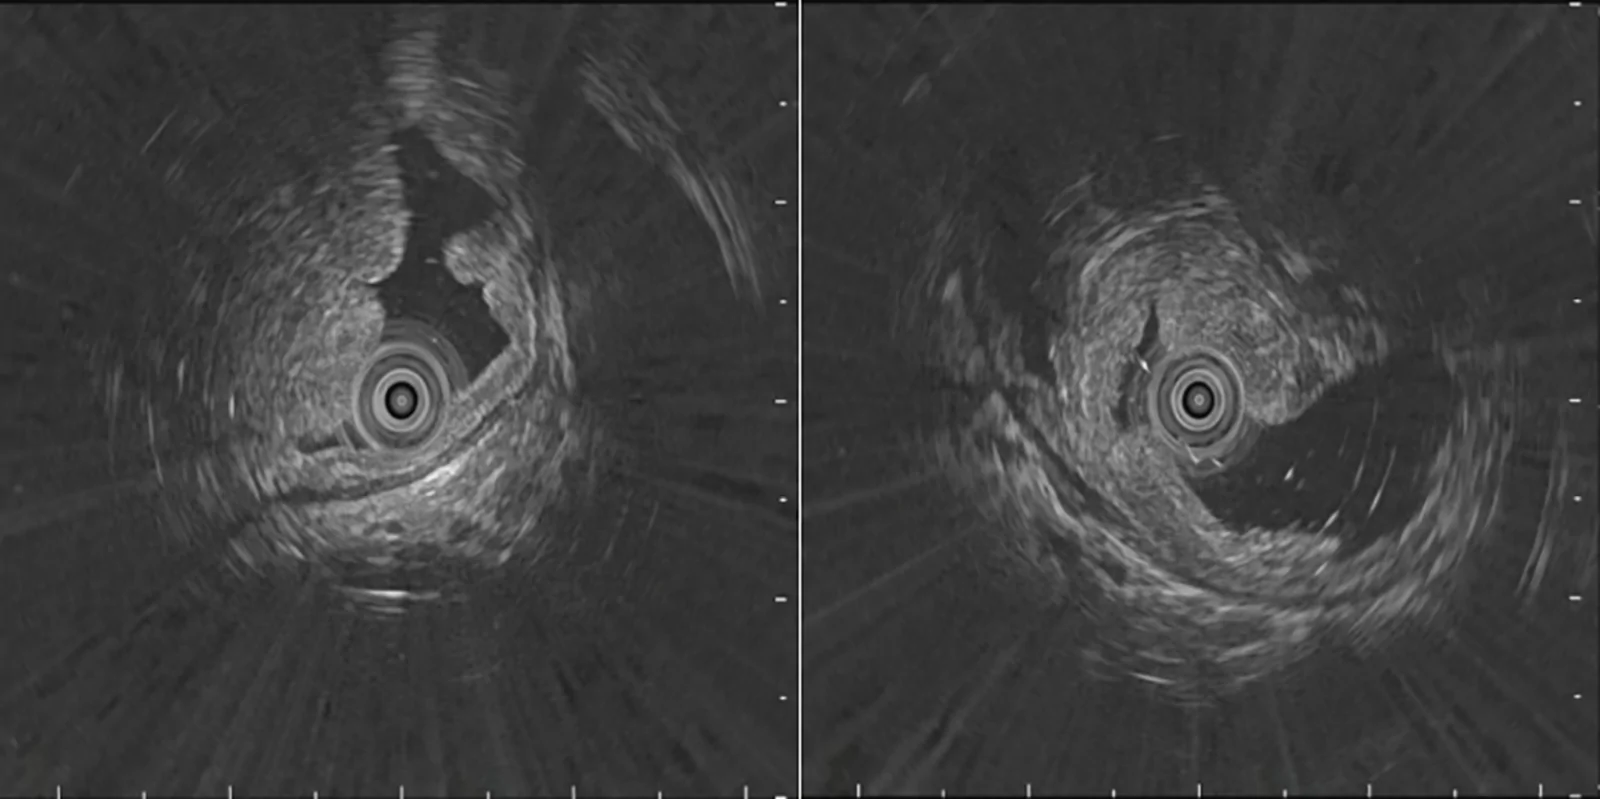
Endoscopic ultrasonography (EUS) suggests that the elevated lesion of the duodenum originates from the mucosal layer and invades the submucosal layer, with no invasion of the biliary and pancreatic ducts.
